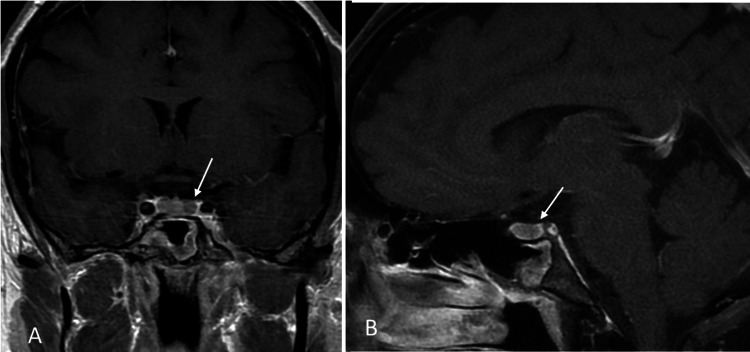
Coronal and sagittal post-contrast MRI (A and B) demonstrate a small hypointense non-enhancing lesion in the pituitary (white arrows) representing a small microadenoma in the patient with MEN1 syndrome
